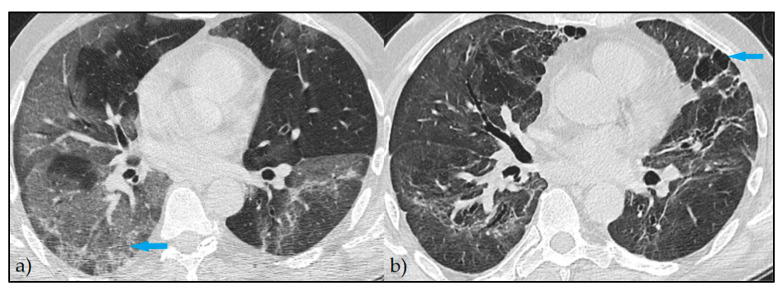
Persistent HRCT findings—Axial HRCT images: (a) extensive areas of GGO at the onset of the symptoms (blue arrow); (b) persistent areas of fine GGO with bronchiectasis, pleural traction, and pleural thickening at 3 months evaluation (blue arrow).
